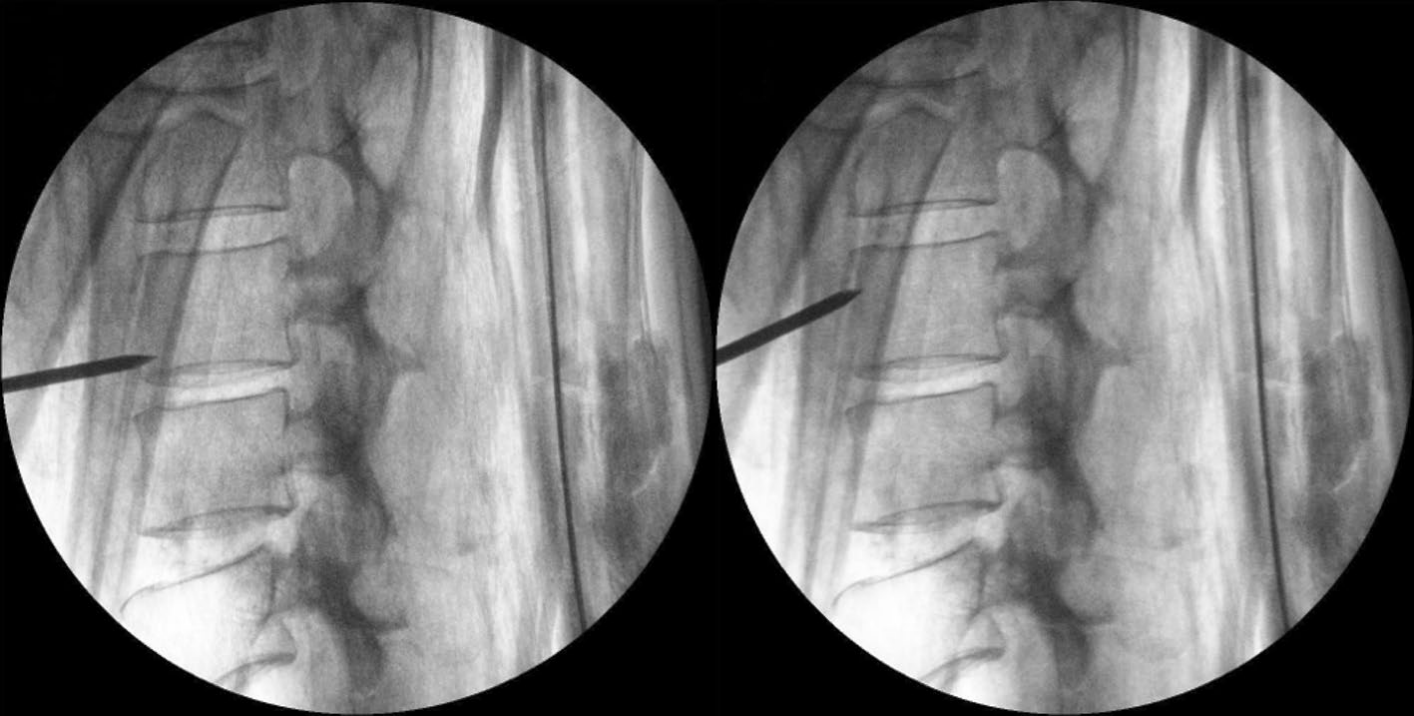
X-ray of the L3 vertebra. Biopsy needle is shown entering the body of L3 at two different angles. Copyright: Robert Loynes, Mark Regan.
